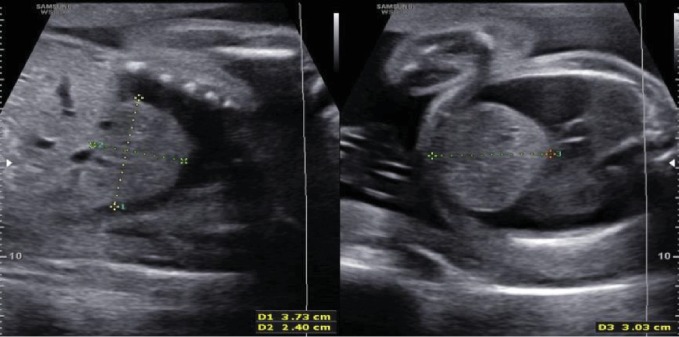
Prenatal ultrasound images of sagittal and transverse sections of fetal thorax, showing large liver lobe protruding into the thoracic cavity.
